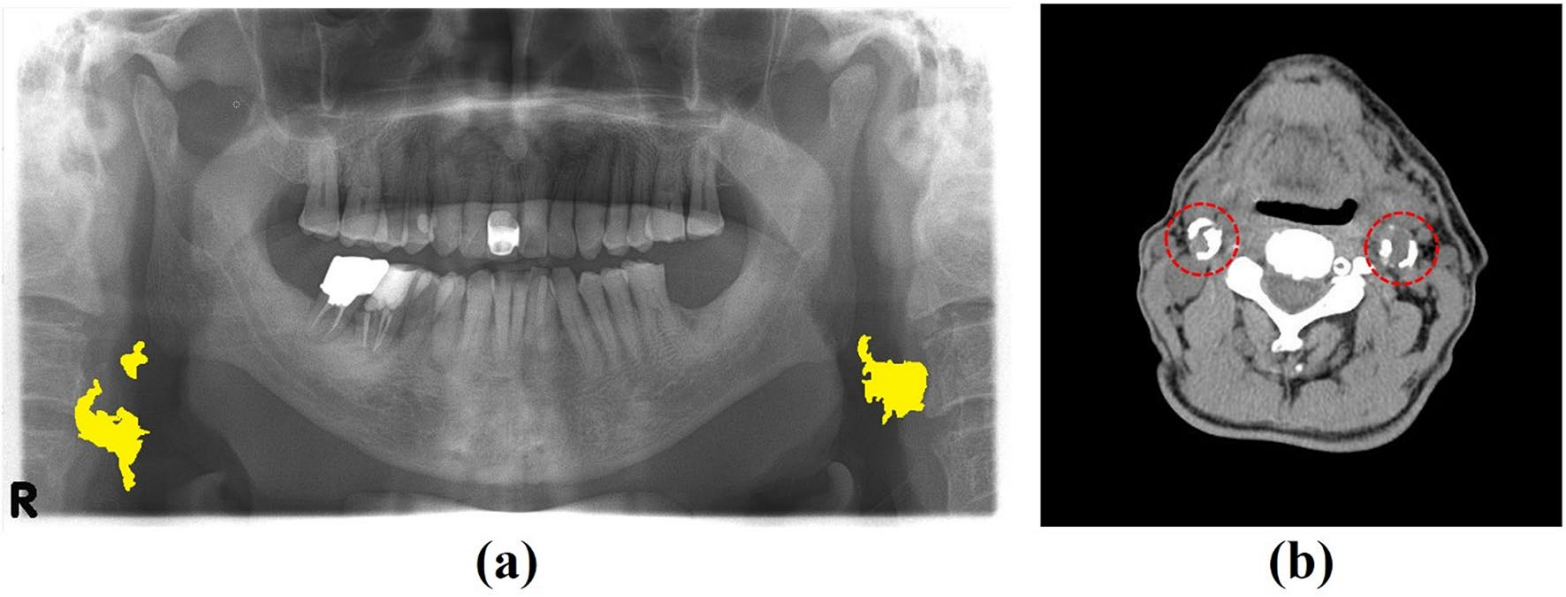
(a) Panoramic radiograph (PR) and (b) CT axial image for a patient. CT images were used as reference for CAC annotation on the PR. Yellow areas indicate carotid artery calcification lesions on the PR. Red dashed circles represent carotid artery calcification on the CT image.
